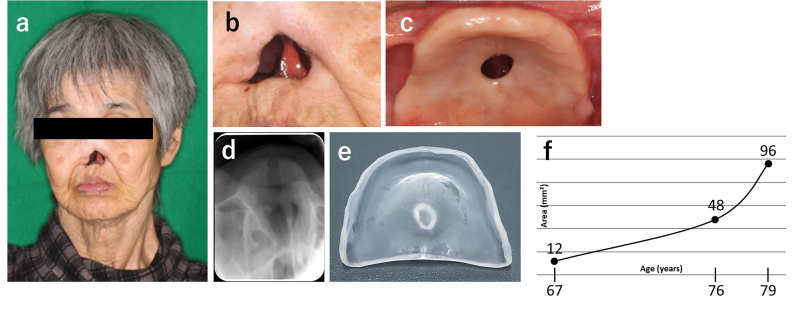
Clinical findings at the 12-year follow-up and the intraoral appliance currently in use (2018 at age 79). (a) Facial photograph; (b) close-up view of the nasal defect; (c) maxillary intraoral photograph; (d) maxillary occlusal radiograph; (e) palatal obturator; (f) Size of the palatal perforation (y-axis: area; x-axis: age).
